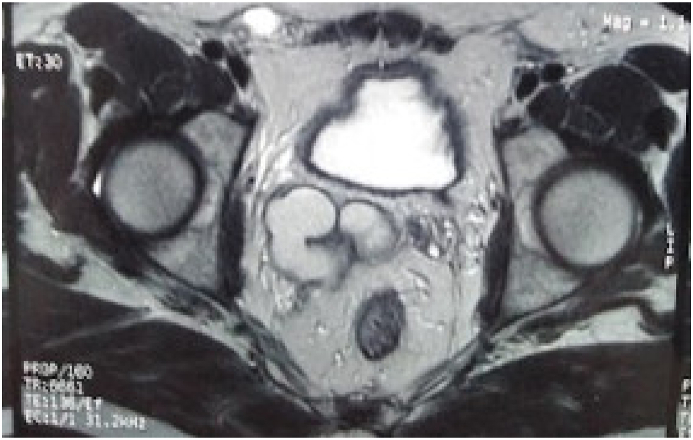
MRI showing the cyst of the right seminal vesicle.
